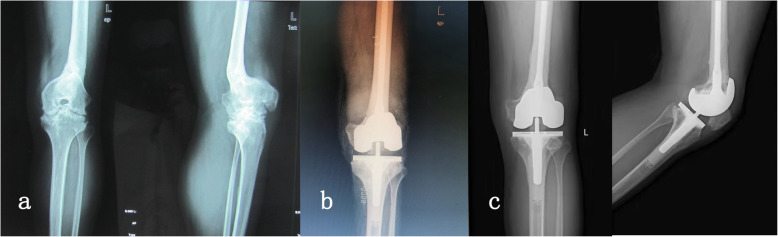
A 37-year-old man (case 1) with recurvatum deformity on femur was treated with one-stage TKA and femoral osteotomy using a constrained condylar knee. a Anteroposterior view and lateral view before surgery. b X-ray at the first day after surgery showing an asymmetrical gap. c Long-term X-ray (91 months postoperatively) showing a stable component and no sign of pronounced wear on tibial insert
